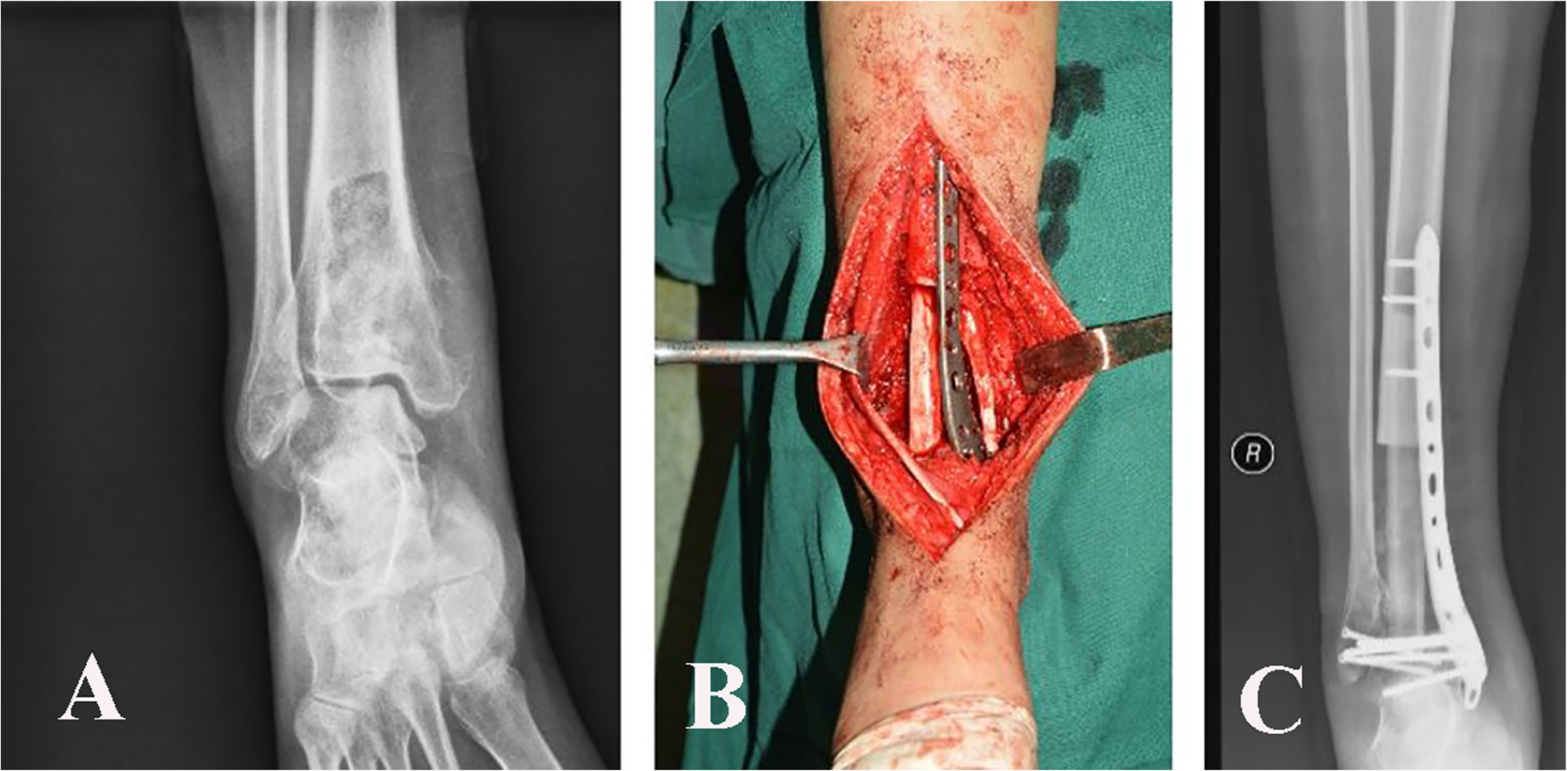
a Preoperative X-ray film shows osteosarcoma of distal tibia. b Intraoperative photo shows that a non-vascularized fibula transfer harvested from unaffected limb restore the tibial continuity, which parallels to the ipsilateral fibula. Fixation was carried out by plate and screws. The talus and ipsilateral fibula were fused. c Postoperative X-ray film
